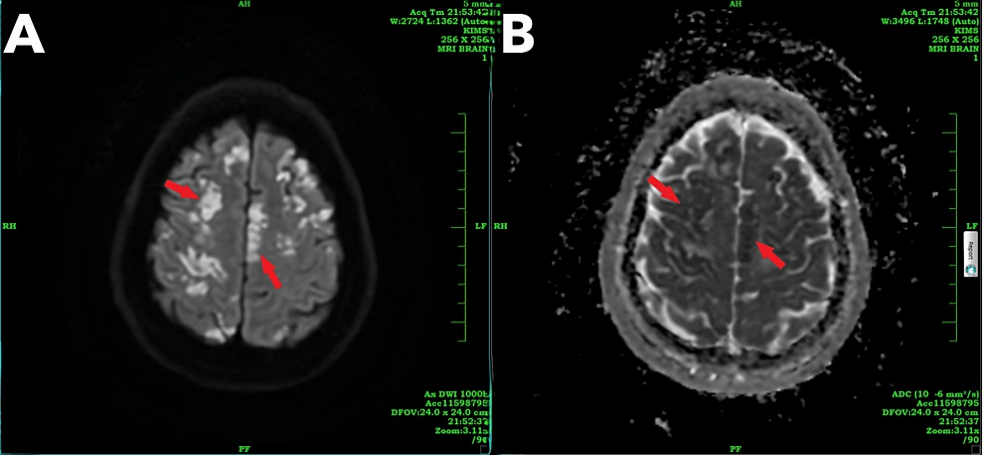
MRI brain showing (A) DWI and (B) ADC The red arrows indicate multiple ill-defined areas of hyperintensity on (A) DWI with corresponding hypointensity on (B) ADC, suggesting restricted diffusion noted in the bilateral frontoparietal lobes. ADC, apparent diffusion coefficient; DWI, diffusion-weighted imaging
